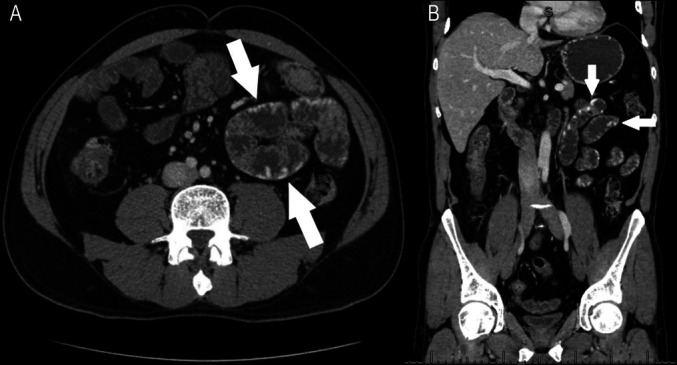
(A) Transverse and (B) coronal section of computed tomography enterography showing varices within the midjejunum (arrows).
